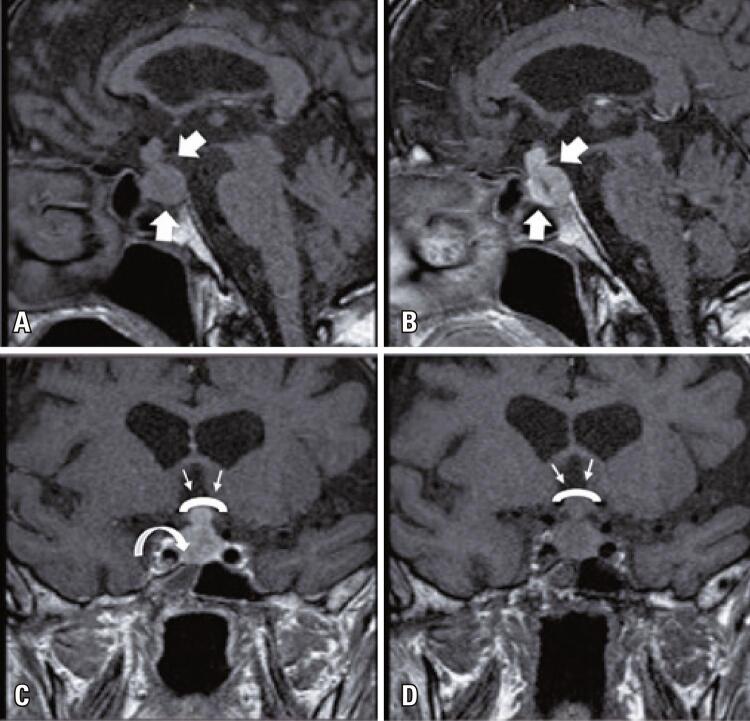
Pituitary magnetic resonance images. (A) precontrast; (B) Postcontrast sagittal T1WI magnetic resonance imaging; (C) Postcontrast; (D) Precontrast coronal T1WI magnetic resonance imaging showing pituitary mass with suprasellar extension (thick white arrows), invasion of the right cavernous sinus (curved white arrow), and optic chiasm compression (short white arrows)
